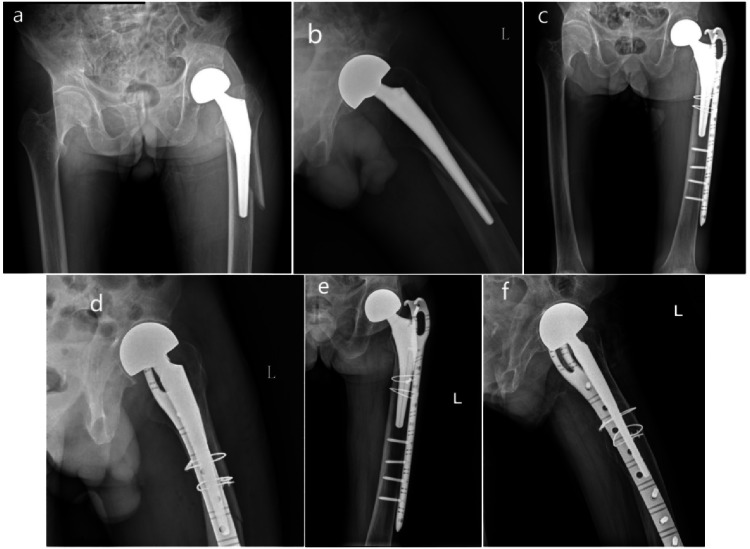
Radiographic examination of a Vancouver B2 PFFs of the left femur in a 83-year-old male (a, b) treated by ORIF. The postoperative radiograph showed an anatomic reduction (c, d). The radiograthic examination taken at follow-up, 4 years later, showed normal fracture healing with stem subsidence about 4 mm (good result, according to Beals and Tower’s criteria) (e, f). At clinical evaluation, the HHS scored 80 points
